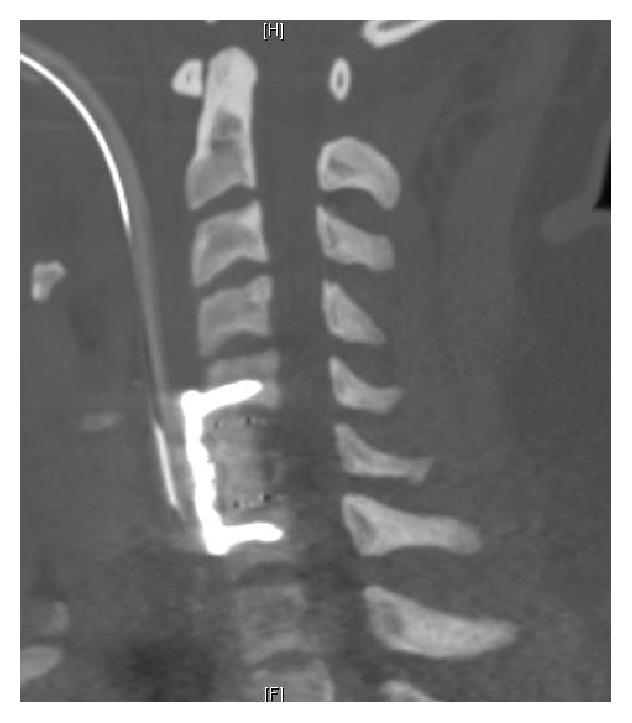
Postsurgical CT scan showing the corpectomy cage and the anterior cervical plate and screws fixation.
